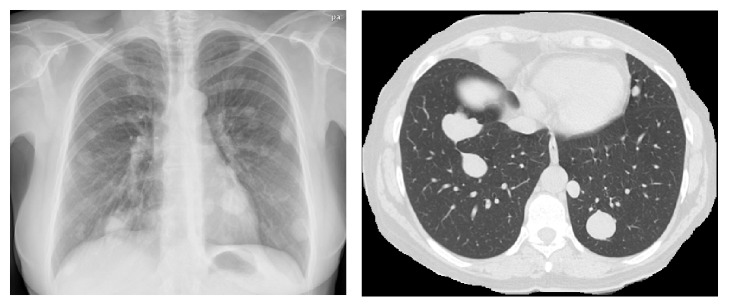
Chest radiography and CT images of patient 2.
